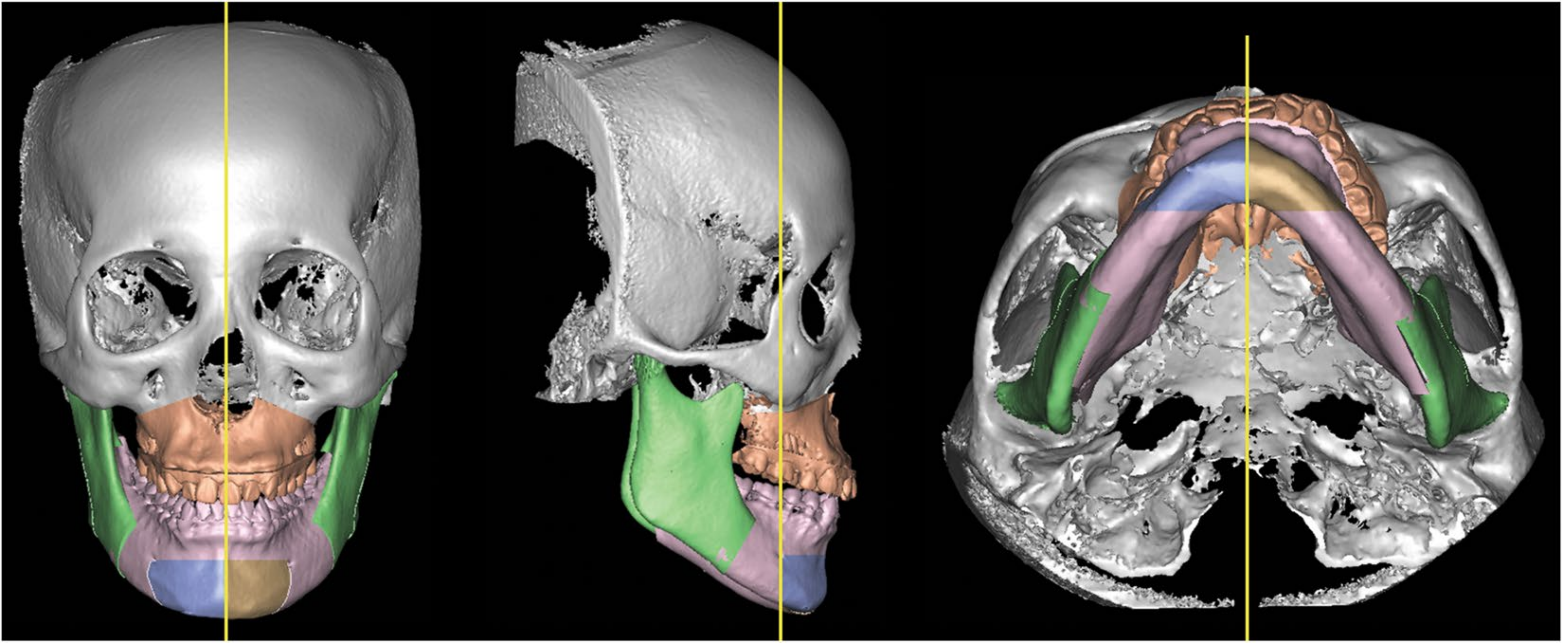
Computed tomography images used for volumetric measurements of jaw symmetry (volumetric discrepancy between right and left mandibular body).
